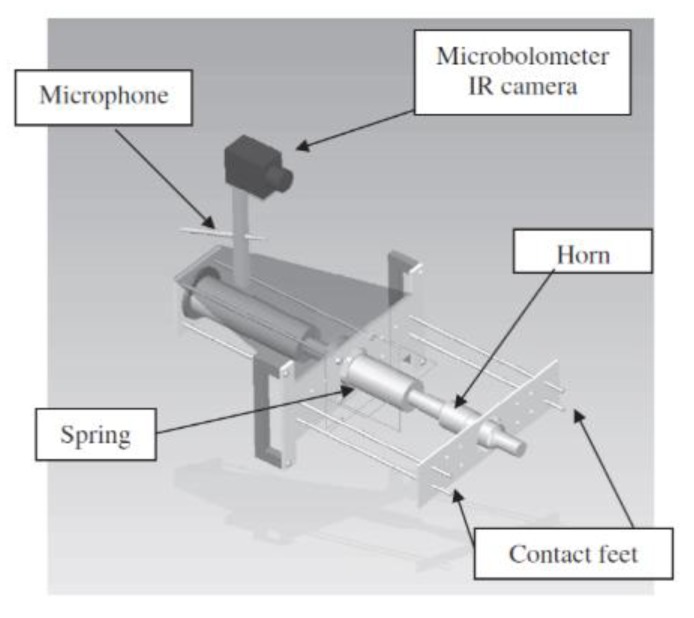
Experimental set-up of ultrasonic stimulated thermography, with permission from [98].
